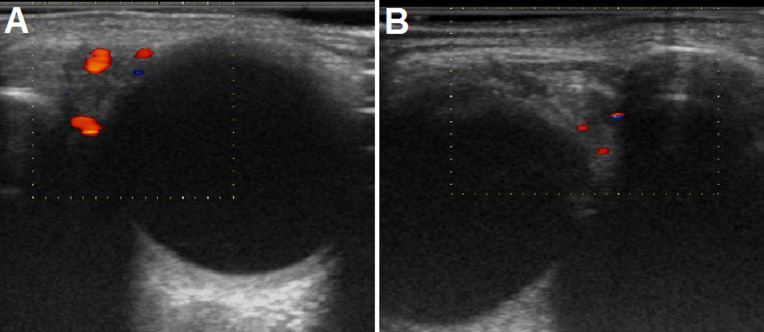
ultrasound of the lacrimal glands after treatment: left (A) and right (B) lacrimal glands decreased in size (left: 9 x 4 mm and right: 8 x 5 mm), and the vascularity and hypoechoic areas disappeared in response to corticosteroid therapy
